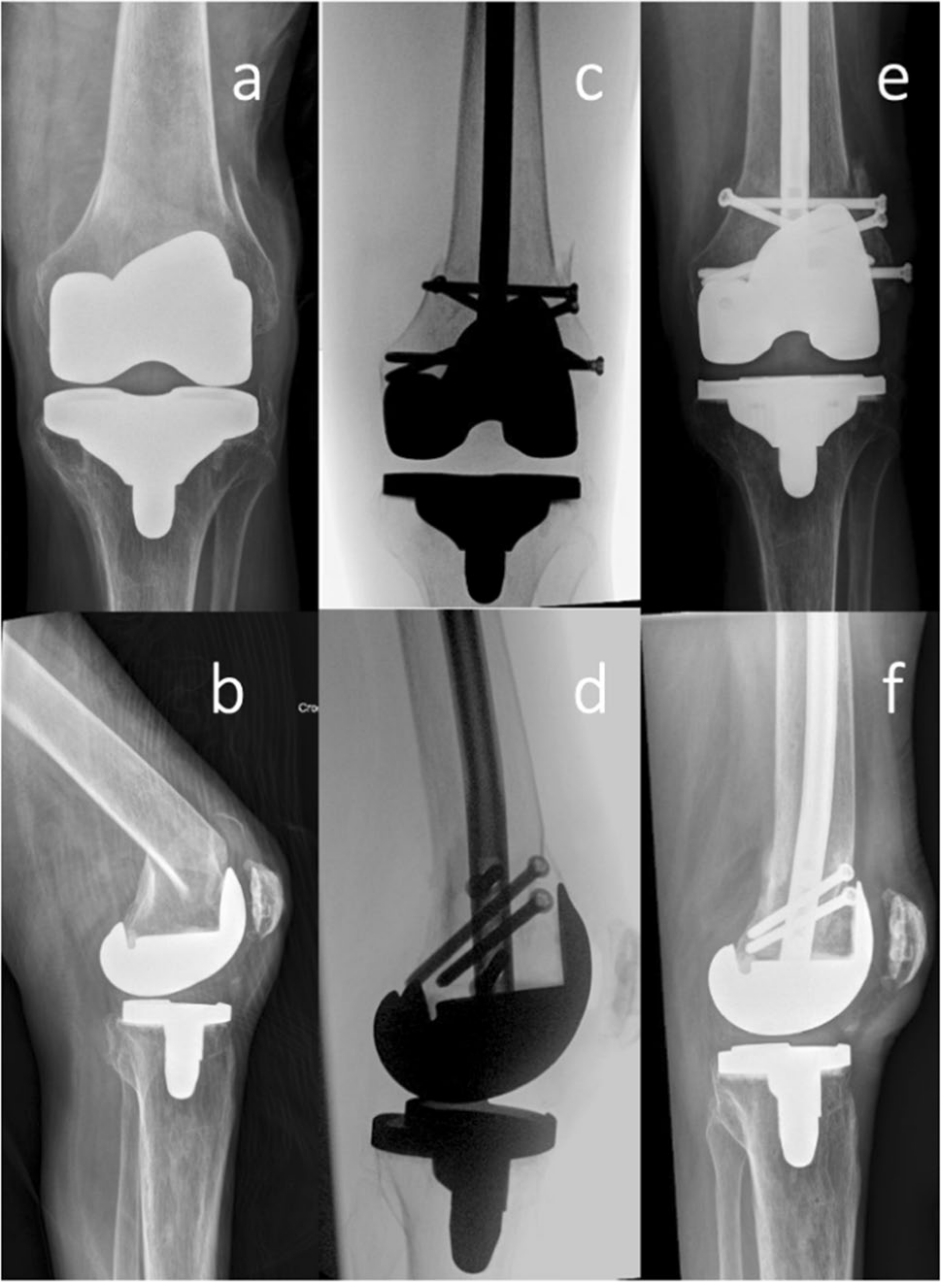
Injury AP (a) and lateral (b) radiographs demonstrating a low periprosthetic distal femur fracture adjacent to a knee arthroplasty component. Intraoperative AP (c) and lateral (d) fluoroscopic images following reduction and surgical fixation using the RFNA. Six-week follow-up AP (e) and lateral (f) radiographs demonstrating unchanged alignment and 3.9 mm distal interlock screw back-out (note the lateral to medial distal-most screw position in image (e)
